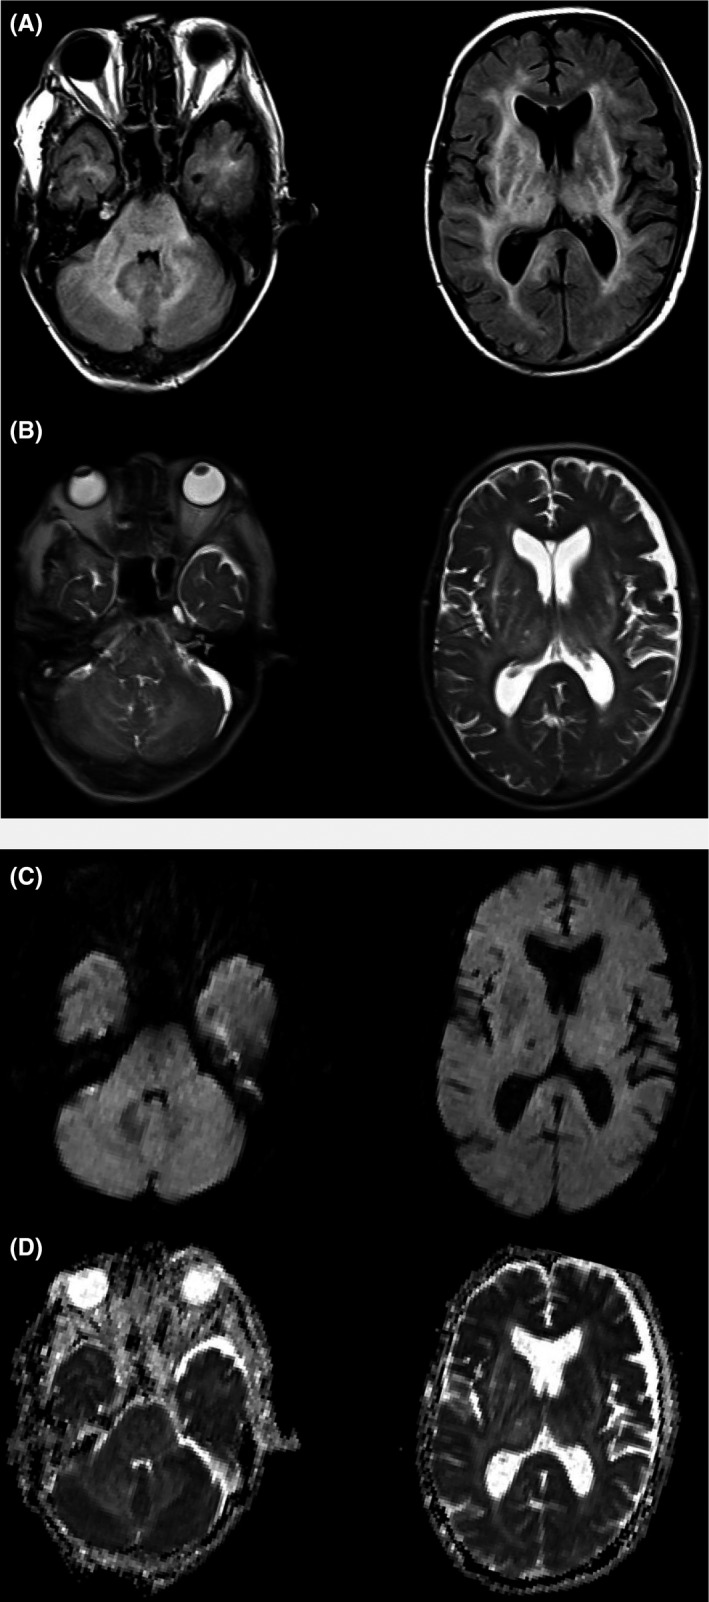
Brain magnetic resonance imaging (MRI) on day 2. T2‐weighted images (A) showed high‐intensity areas in the brainstem, cerebellum, and cerebral white matter (symmetrically). Fluid‐attenuated inversion recovery (FLAIR)images (B) showed the same high‐intensity areas as observed on the T2‐weighted images. There were no apparent abnormally intense areas indicating an acute brain infarction on diffusion‐weighted imaging (DWI) (C) and apparent diffusion coefficient (ADC) maps (D)
